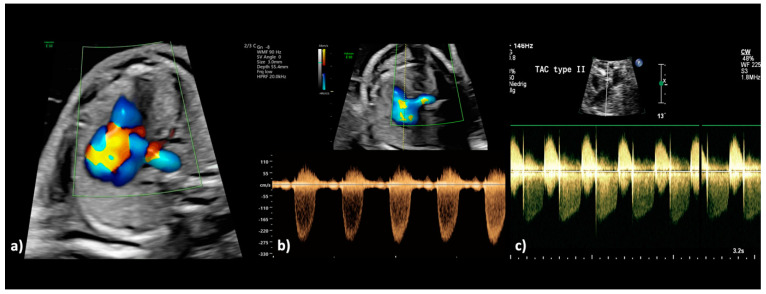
(a–c): Truncus arteriosus type 1 at 22 + 0 weeks. (a) In the color Doppler mode, the filling of the common arterial trunk from both ventricles and the confluent origin of both pulmonary arteries from the posterior side of the aorta is visualized (aortic dominance), as well as aliasing and high variance as indications of disturbed blood flow due to stenosis of the truncal valve. (b) The spectral Doppler examination confirms the presence of a stenotic truncal valve by a high maximum velocity (peak systolic velocity: 3.0 m/s; maximum pressure gradient (ΔPmax: 36 mm Hg) and wide variation of the blood flow. (c) In another fetus with truncus arteriosus type A2 at 34 + 1 weeks, there is both a relevant stenosis and a severe regurgitation of the truncal valve; during systole, the tubular antegrade flow with a peak velocity of 2.40 m/s can be recognized; during diastole, the insufficiency of the truncal valve with a peak velocity of 3.30 m/s can be recognized.
